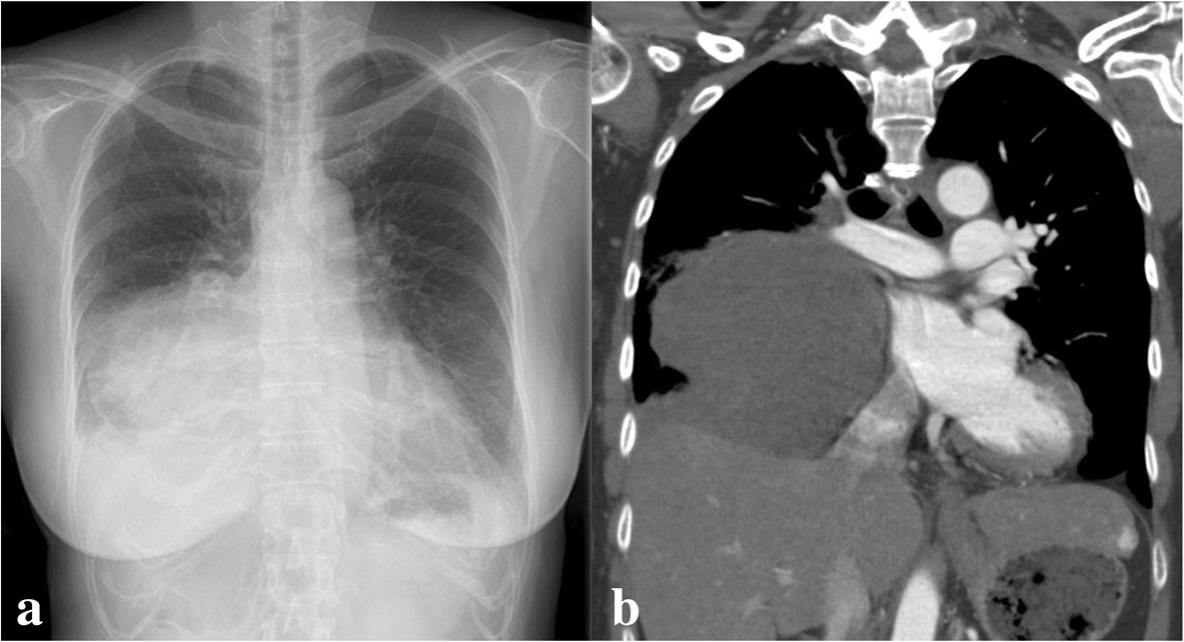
Second chest radiography (a) shows an enlarged mass with an irregular shape. Enhanced computed tomography (b) after the admission demonstrates occlusion of the right inferior pulmonary vein due to tumor compression
